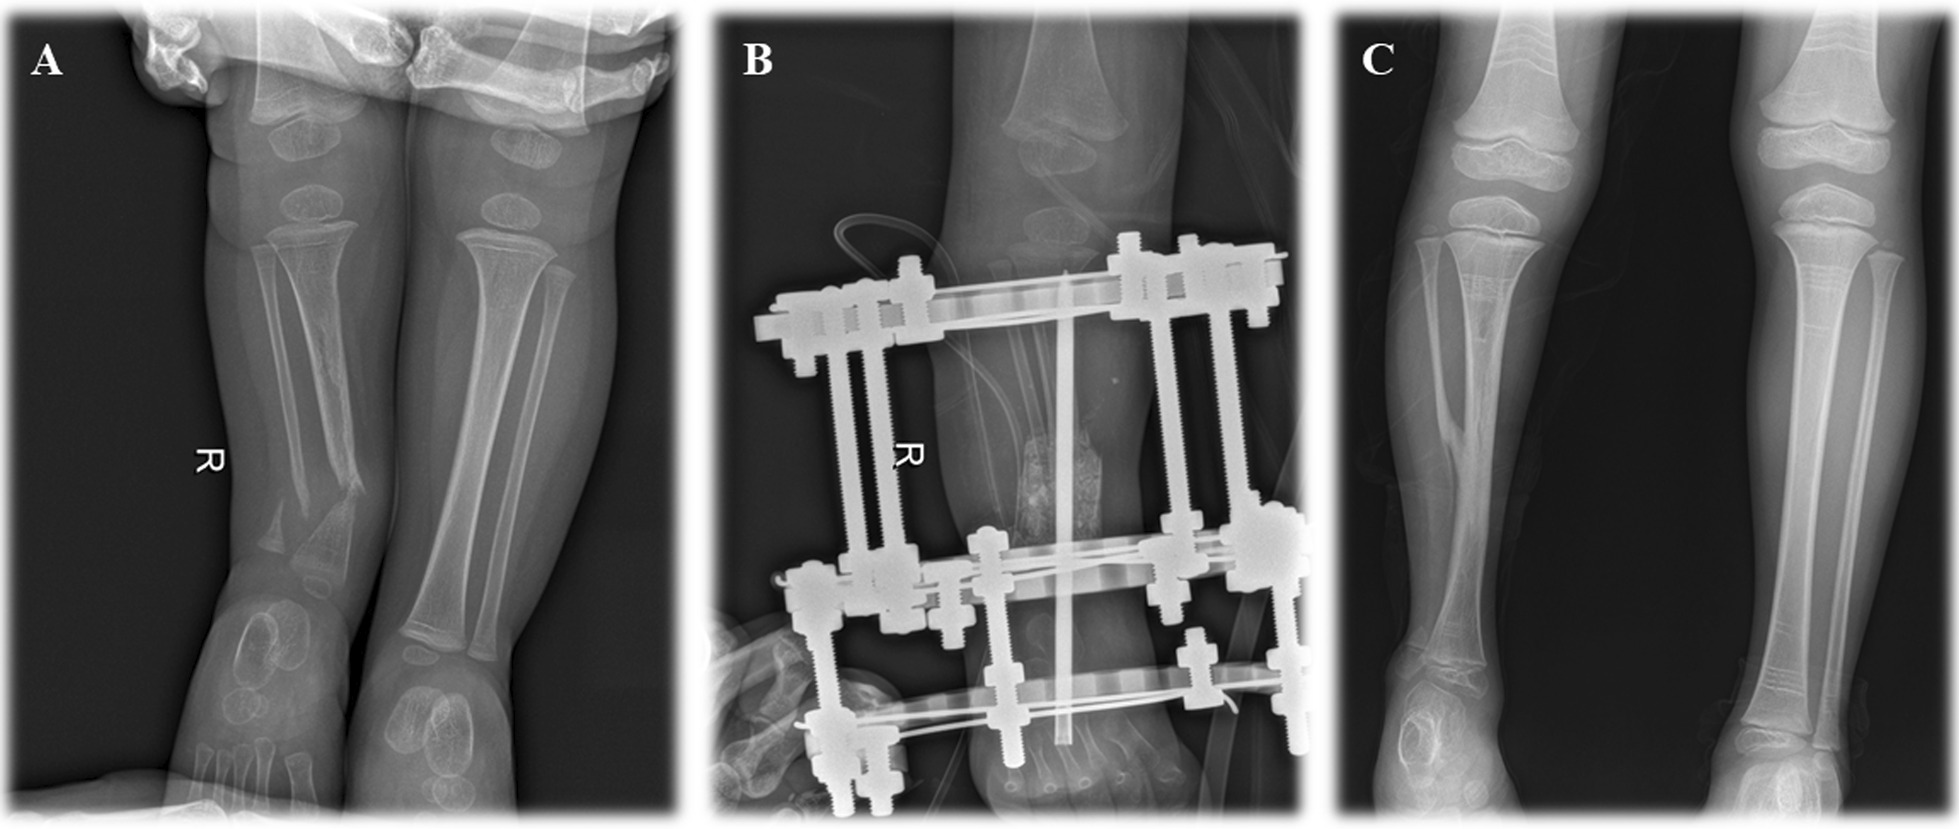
Preoperative and postoperative X-rays of combined surgery with cross-union. A preoperative X-ray; B postoperative X-ray within 7 days; C postoperative X-ray after primary union
